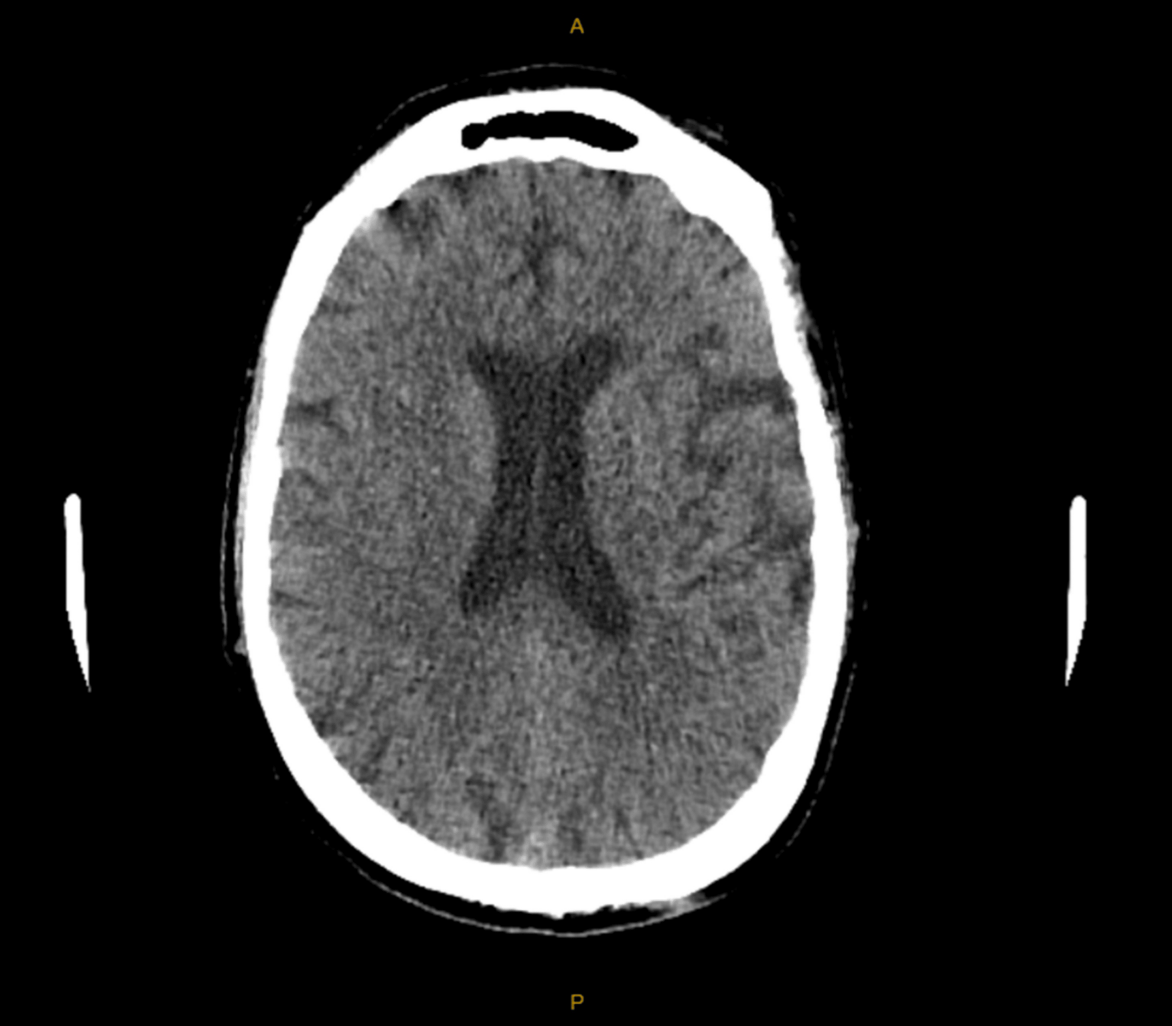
CT Brain Scan With Contrast Day 1 - Normal Study CT: computerised tomography
